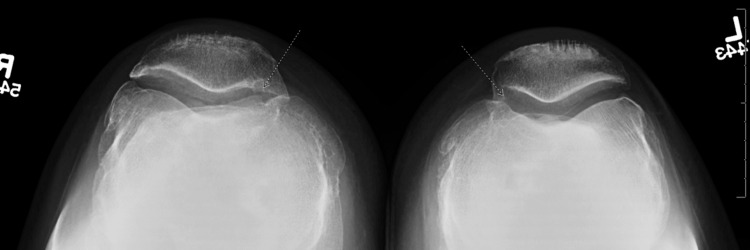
Preoperative radiographs. Bilateral sunrise view of the knees showing degenerative changes. Arrows show bilateral joint space narrowing.
